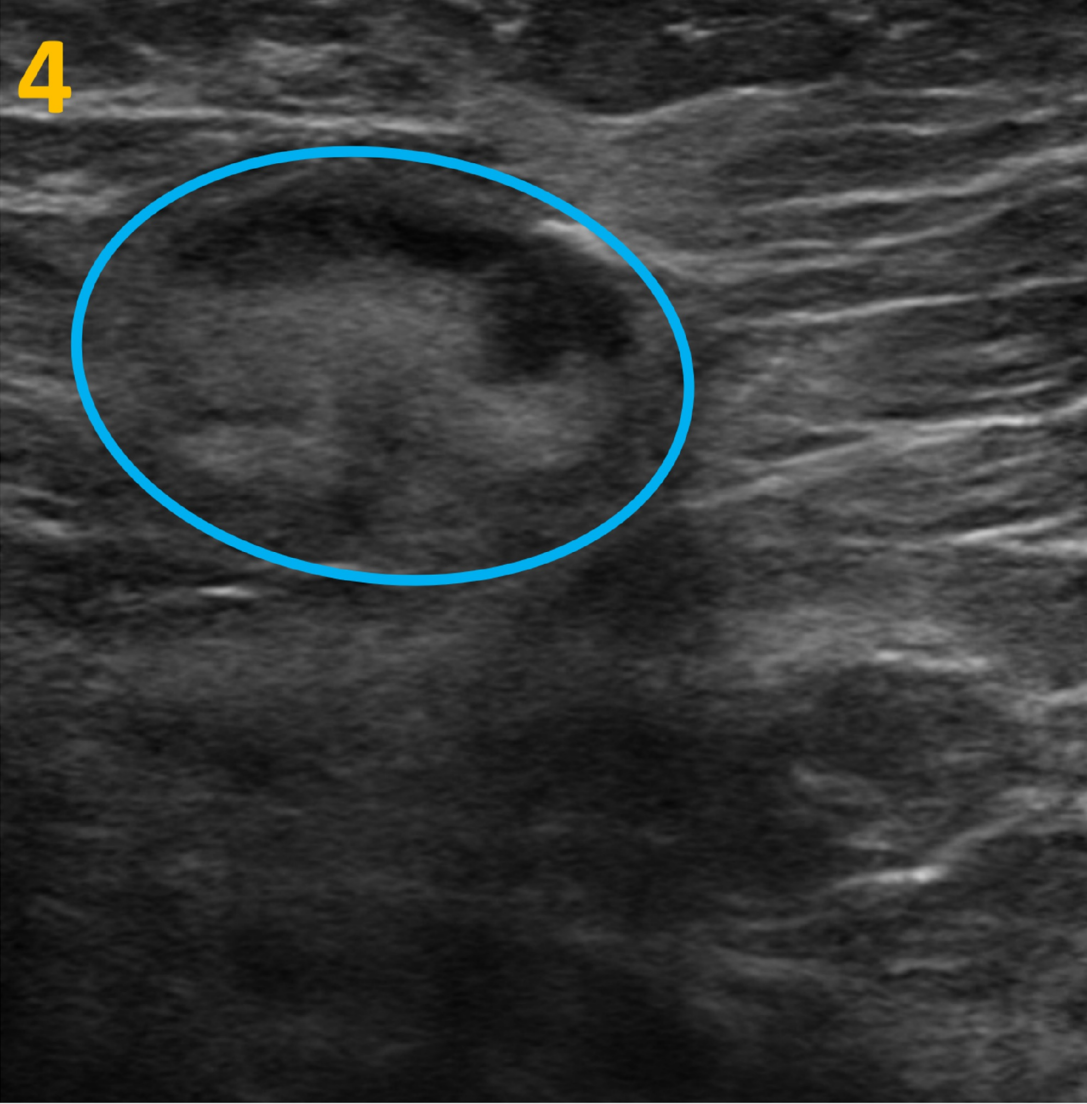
Targeted Ultrasound of the Right Axilla This ultrasound image demonstrates a morphologically abnormal lymph node measuring 15 mm with eccentric cortical thickening in the right axilla (blue circle).
